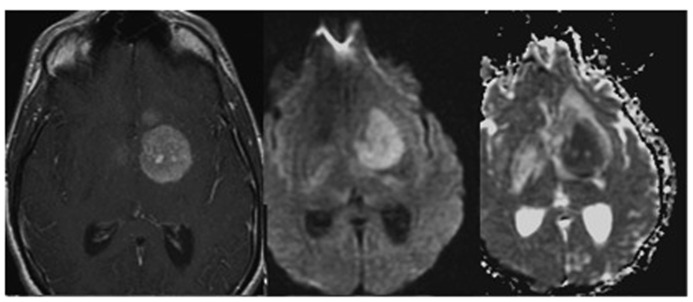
T1-weighted image of a glioma following contrast enhancement using a gadolinium-based contrast agent (left). Diffusion weighted images DWI (middle), and apparent diffusion coefficient map ADC (right) of the same tumor patient. Adapted from Young (2007), reproduced with permission.
